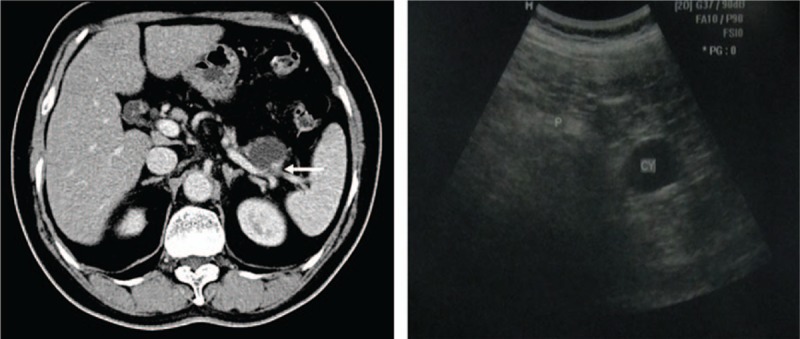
Preoperative CT and ultrasonography scans of our patient, with an ill-defined cystic lesion located in the pancreatic tail, and the arrow points the mural nodules. The tumor was 3.3 × 2.6 cm in CT scans and 3.4 × 2.5 cm in ultrasonography. CT = computed tomography.
